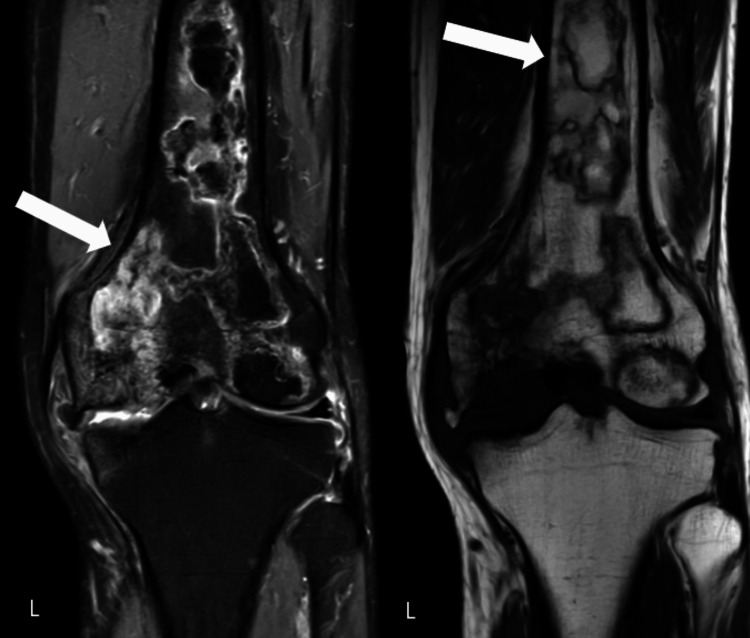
Magnetic resonance imaging of left knee This image shows bone edema and multiple bony infarcts in both condyles and the lower third of the left femur (marked with arrows).
